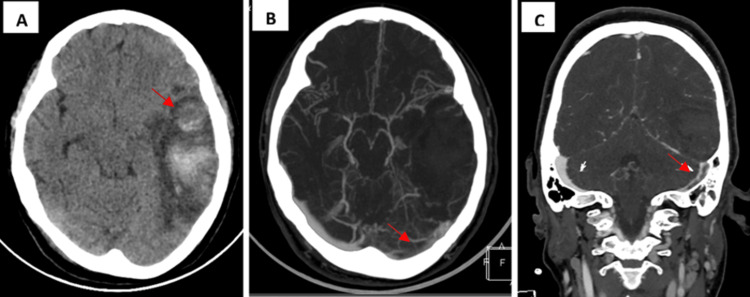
Follow-up CT scans eight days after admission and CT angiography. Follow-up CT scans eight days after admission showed a reduction of the dimensions of the left parietotemporal intraparenchymal hematoma (red arrow) (A). CT angiography showed a partially recanalized left transverse sinus (red arrow) (B, C). CT: computed tomography.
